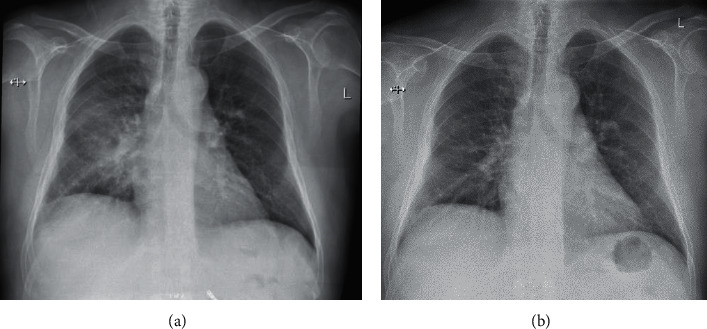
Chest X-ray showing right lower zone non-homogenous opacity with air bronchogram (a). Then, there was remarkable improvement 6 months later (b).
